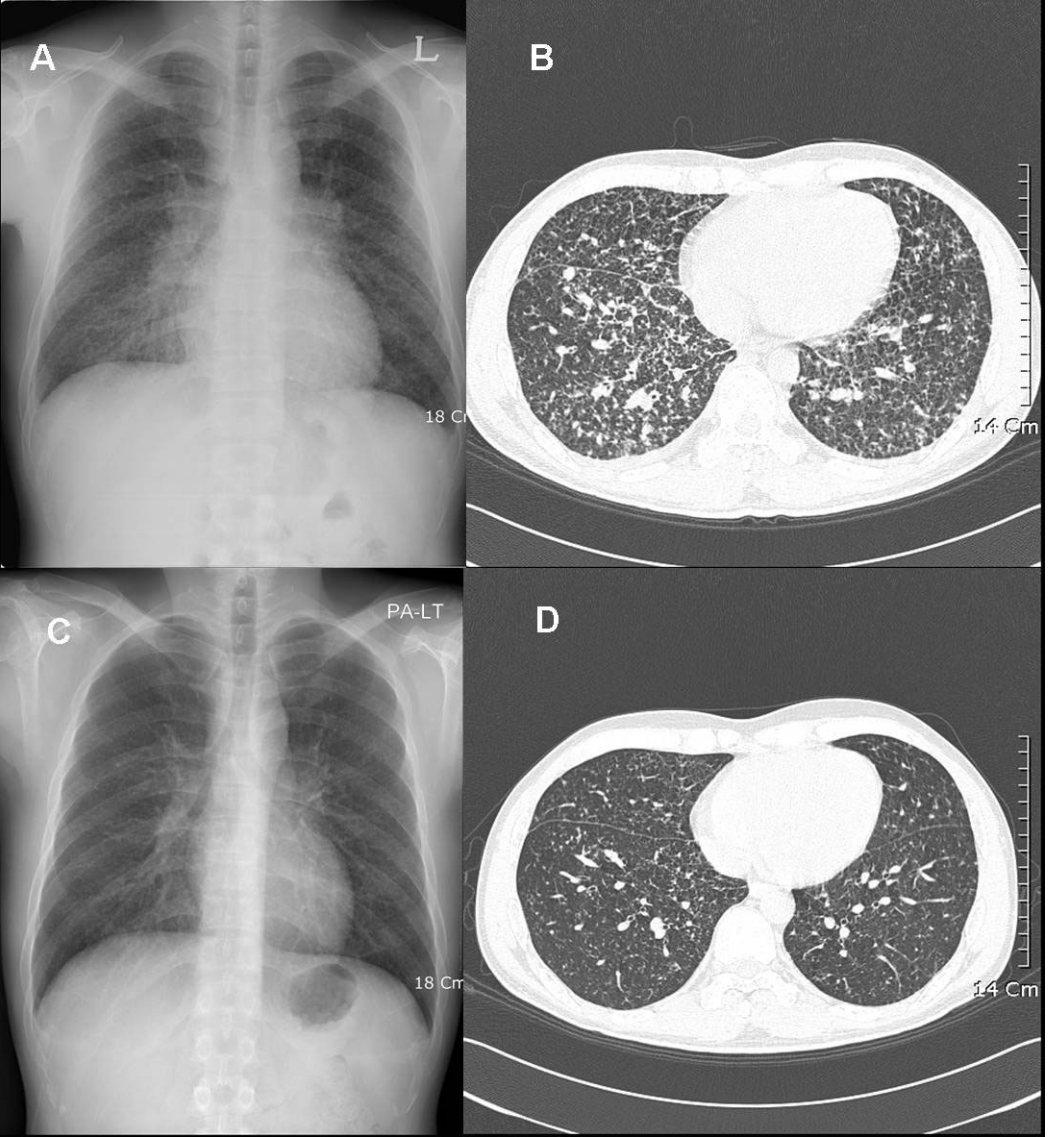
Chest X-ray (CXR) and high-resolution computed tomography (CT) findings on admission, and again at five months following combined treatment. Top (on admission): (A) Posterior-anterior chest radiograph (P-A CXR) shows multiple tiny reticular nodules on both lungs; (B) Tiny centrilobular and subpleural nodules are evident in both lungs on the chest CT. Bottom (five months after combined treatment): (C) A follow-up CXR shows improvement in the multiple reticular nodules; (D) A follow-up CT scan reveals the improvement of the residual nodules.
